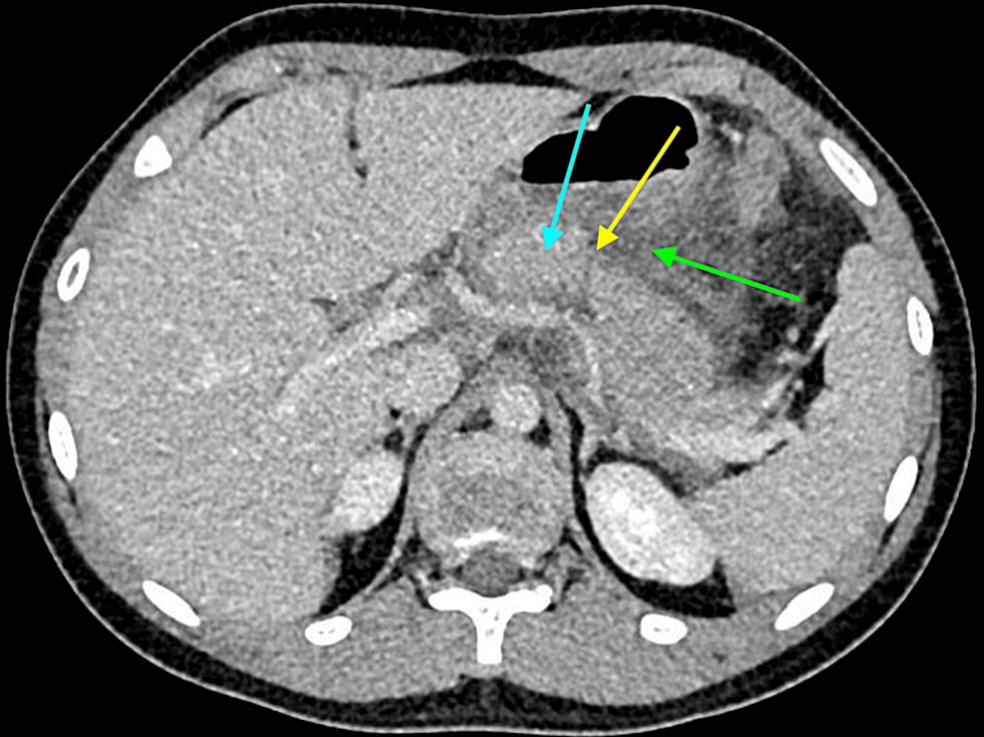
Abdominal CT scan with intravenous contrast. Diffuse inflammation of the pancreas (blue arrow) with the presence of a peripancreatic fluid collection (green arrow). Possible rupture of the pancreatic parenchyma (yellow arrow).
